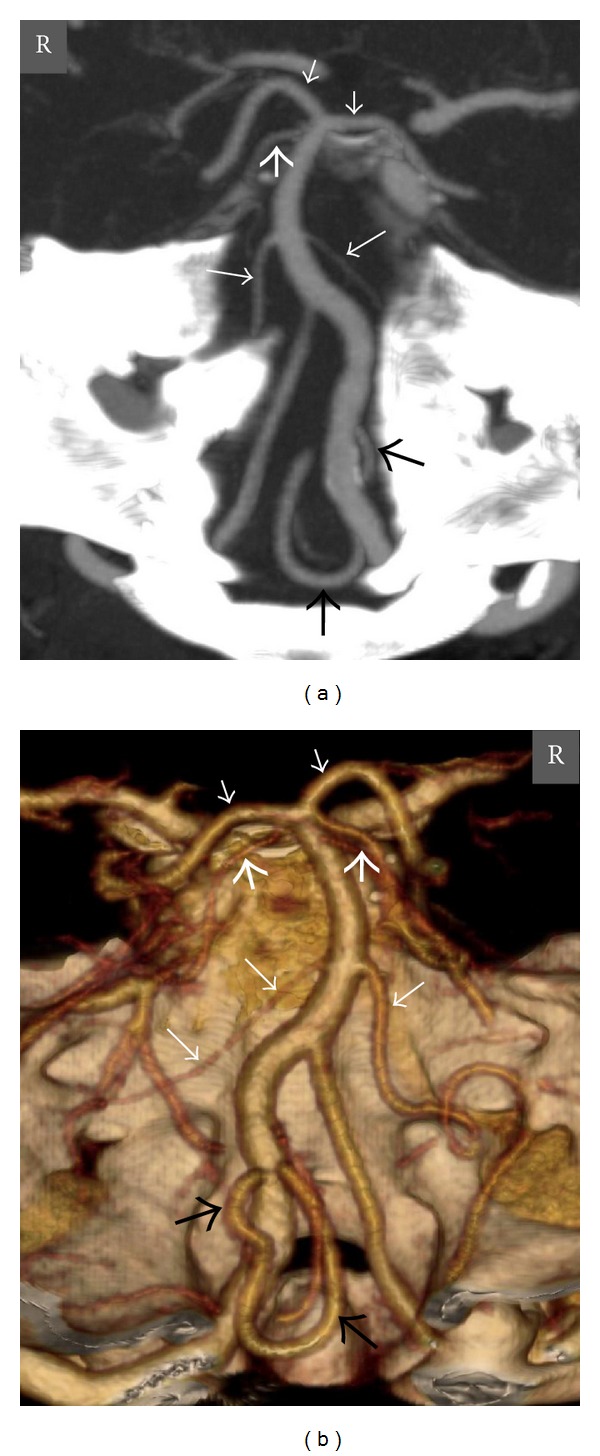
Oblique coronal view MIP (a) and posteroanterior view VR (b) computed tomography (CT) angiography images show absence of the right PICA and well-developed right AICA and left PICA in a 54-year-old man. The images also depict a dominant left vertebral artery. *Thick long arrow *= left PICA, *thin long arrows *= right and left AICAs, *thick short arrows *= right and left SCAs, *thin short arrows *= right and left PCAs, and *R* = right.
